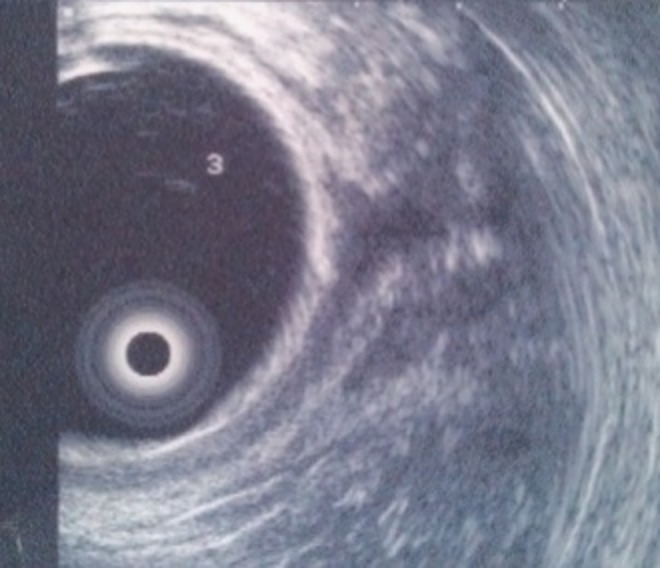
Endoscopic ultrasound image of the fistula. The fistula tract is depicted in the 12th hour of the rectum, suggesting a suprasphincteric fistula 3 cm above the anal verge.
